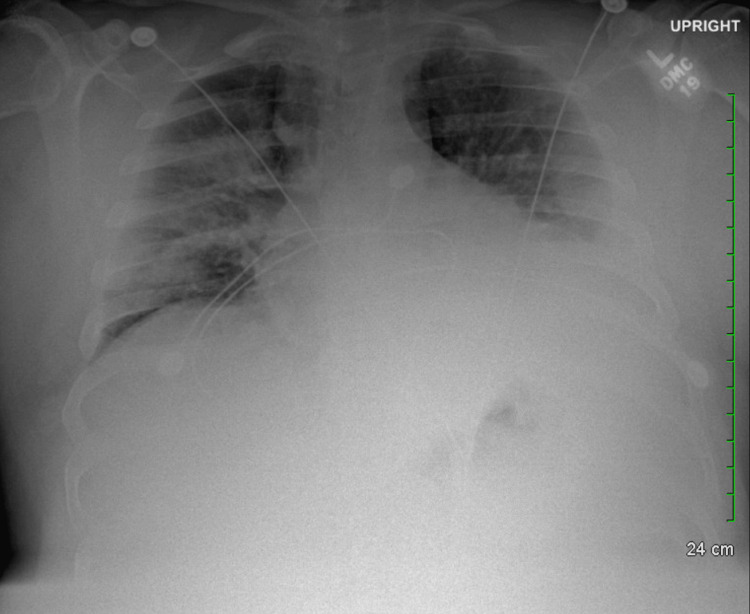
Initial chest X-ray in emergency department
